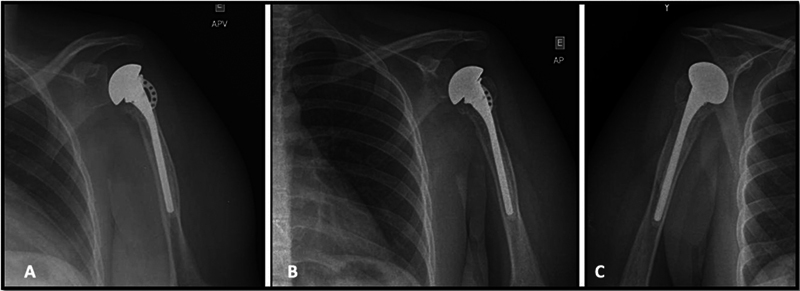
Immediate postoperative radiograph: (A) true anteroposterior view; (B) anteroposterior view; (C) oblique view.
